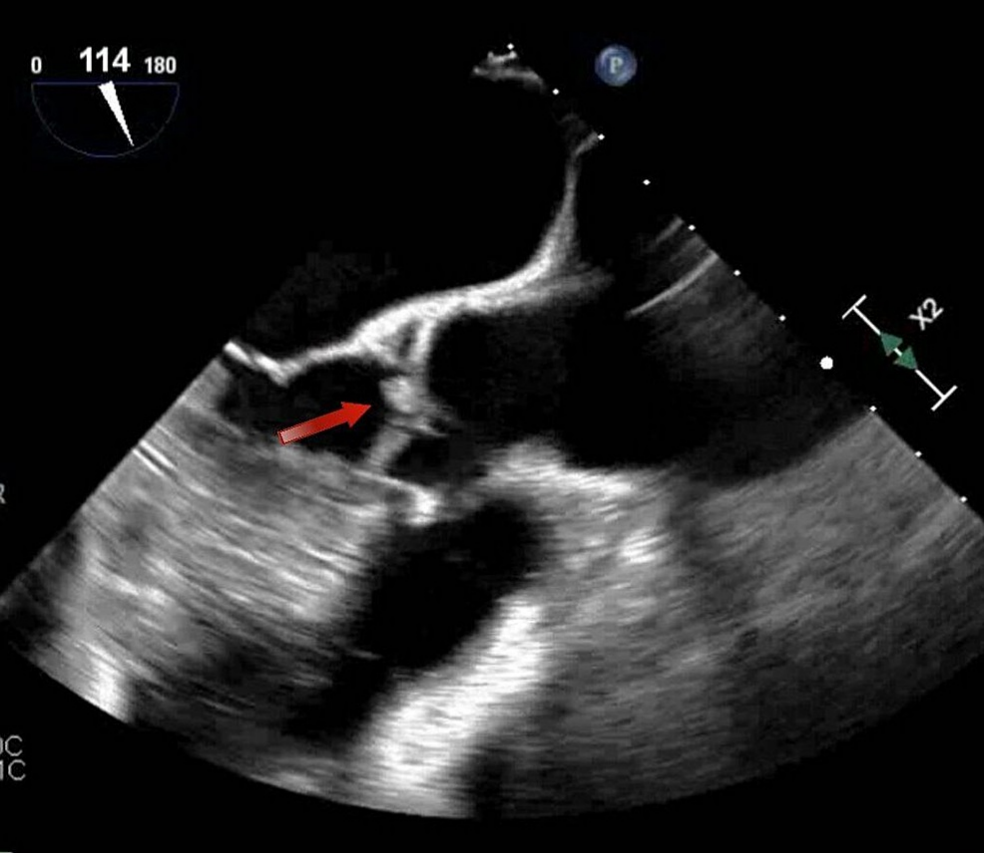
Transesophageal echocardiogram (TEE) showing vegetation attached on the ventricular side of aortic valve
